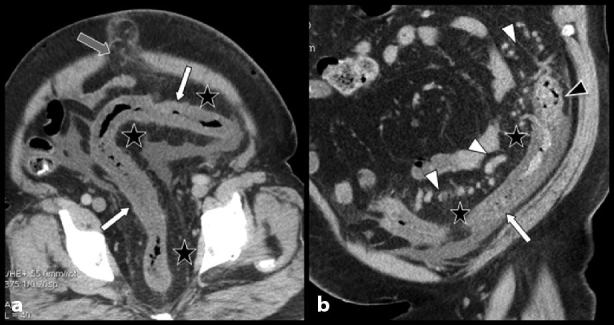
Contrast-enhanced abdominal CT images. Oblique (a) and coronal oblique (b) multiplanar reconstructions show severe but homogeneously circumferential hypodense thickening of a rather long segment of the left colon extending from the descending colon to the rectosigmoid junction (white arrows) and fat stranding of the umbilical hernia (grey arrow). There is a sharp cutoff of the thickening at the level of the left phrenicocolic ligament. Diffuse congestive fat stranding is associated with the mesosigmoid and the greater omentum (black stars). Unusually large and serpiginous varicosities are found not only in the mesosigmoid fat (white arrowheads on b) but also in the colonic wall (black arrowhead on b). Ascites are visible in the sigmoid and the right iliac fossa.
